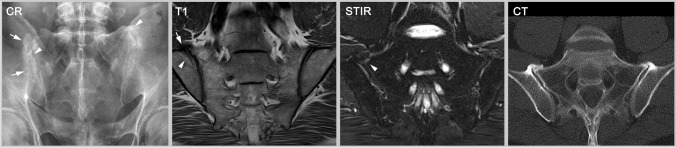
Fifty-six-year-old patient with osteoarthritis. Radiography can easily be falsely judged as positive because osteophytes can mimic erosion and sclerosis (arrows) and even partial ankylosis (arrowheads) when bone proliferation is superimposed over the joint but is in fact external to the articular surface and is around the joint. On MRI, it may be harder to see the bridging osteophytes (arrow), especially compared to CT. Also, osteoarthritis can show small bone marrow lesions with fat deposition (arrowhead in T1) or edema (arrowhead in STIR), especially near osteophytes. In this case, the radiography is ‘false-positive’ but the MRI is ‘true-negative’ for sacroiliitis
